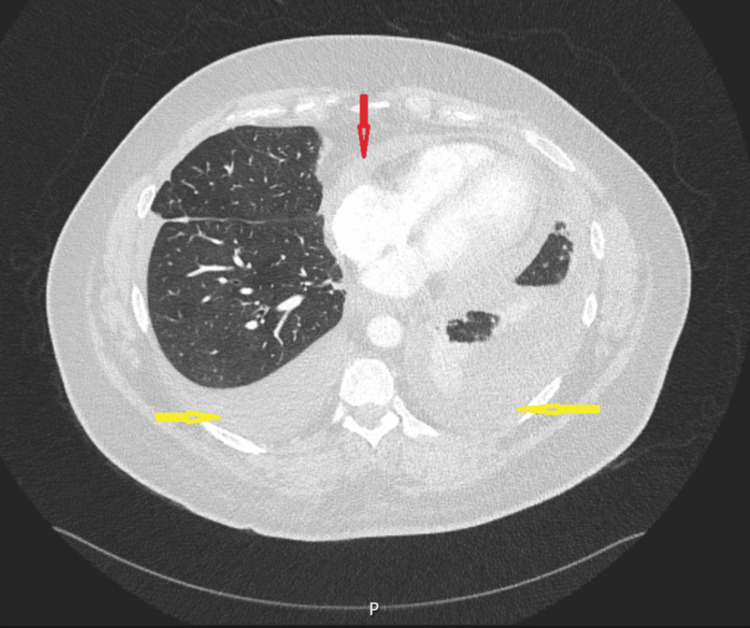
CT scan of the thorax showing bilateral pleural effusions (yellow arrows) and pericardial effusion (red arrow)
